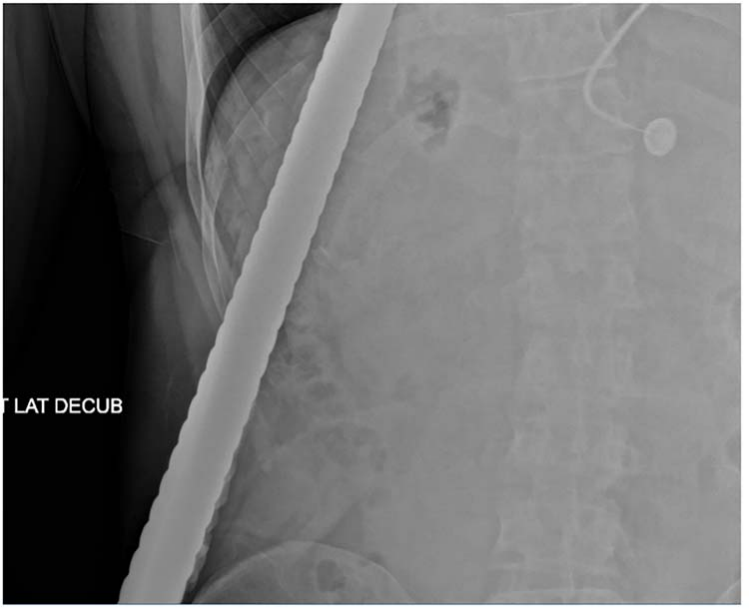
Abdominal X-ray demonstrating the rebar trajectory into the left anterior chest; the path is distorted secondary to patient positioning during imaging.
